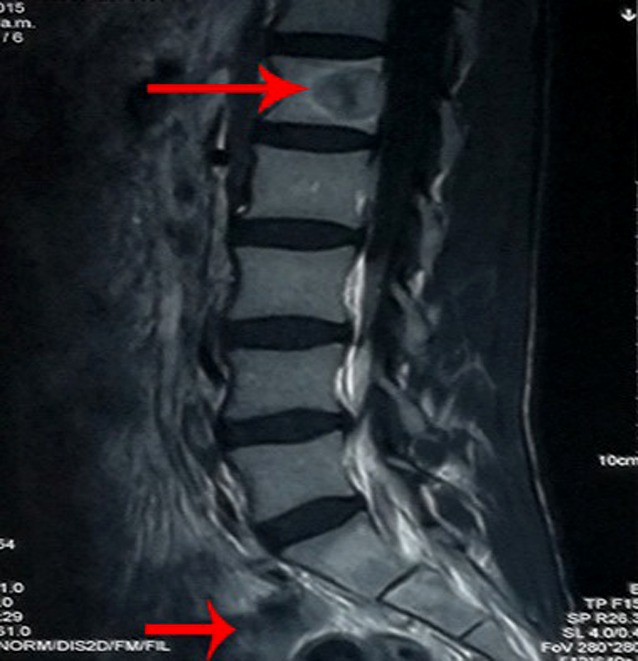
Preoperative magnetic resonance imaging of L1 and S1–S2 reveals bone destruction and vertebral abnormalities. *Red arrows* indicate L1 (*upper arrow*) and S1-S2 (*lower arrow*) lesions in MRI
